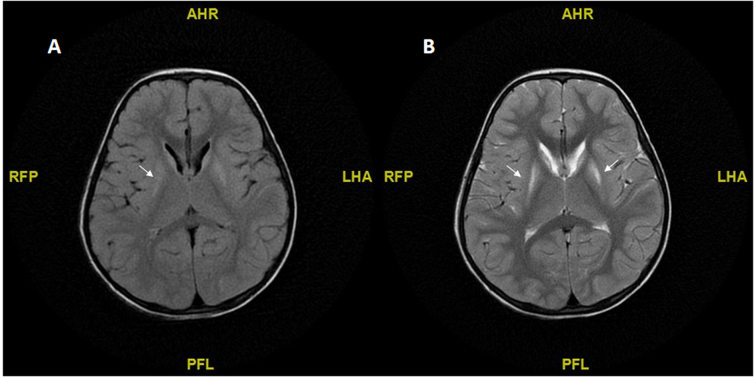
Fig 1a. MRI brain-FLAIR sequence. Fig 1b. MRI brain-T2 sequence Bilateral and symmetrical high signal intensity affecting putamen and caudate nuclei, the later is associated with atrophic changes.
